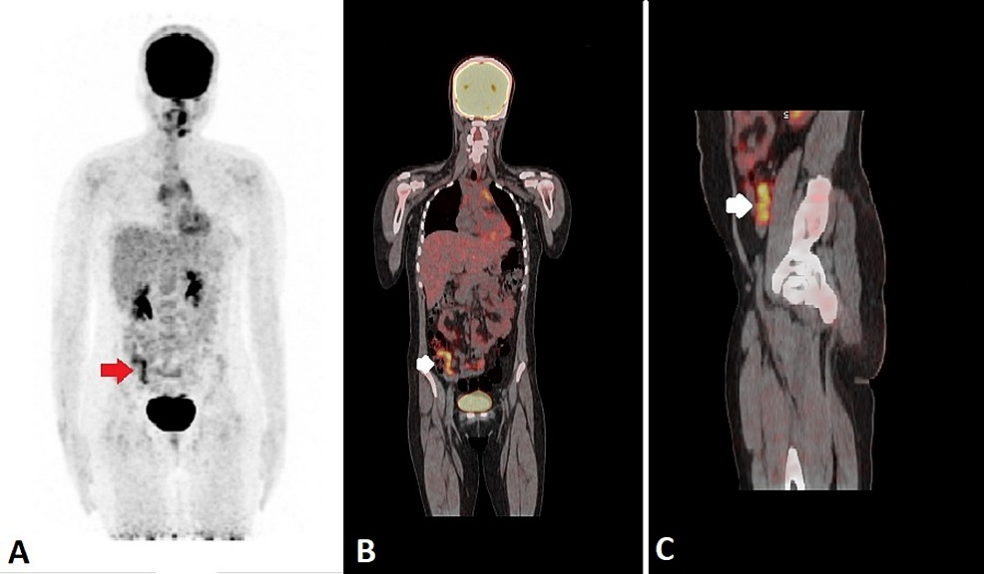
18F-FDG PET/CT: (A) coronal MIP image, (B) coronal fused image and (C) sagittal fused image (A) MIP image shows an 18F-FDG avid finger-like structure (red arrow) in the right iliac region. (B) Coronal fused image shows a hypermetabolic finger-like structure (white arrow) in the right iliac region. (C) Sagittal fused image shows hypermetabolic inflamed appendix (white arrow) lying anterior to the right psoas muscle. MIP: maximum intensity projection 18F-FDG PET/CT: Fluorine-18-Fluorodeoxyglucose Positron Emission Tomography/Computed Tomography 18F-FDG: F-18 fluorodeoxyglucose
